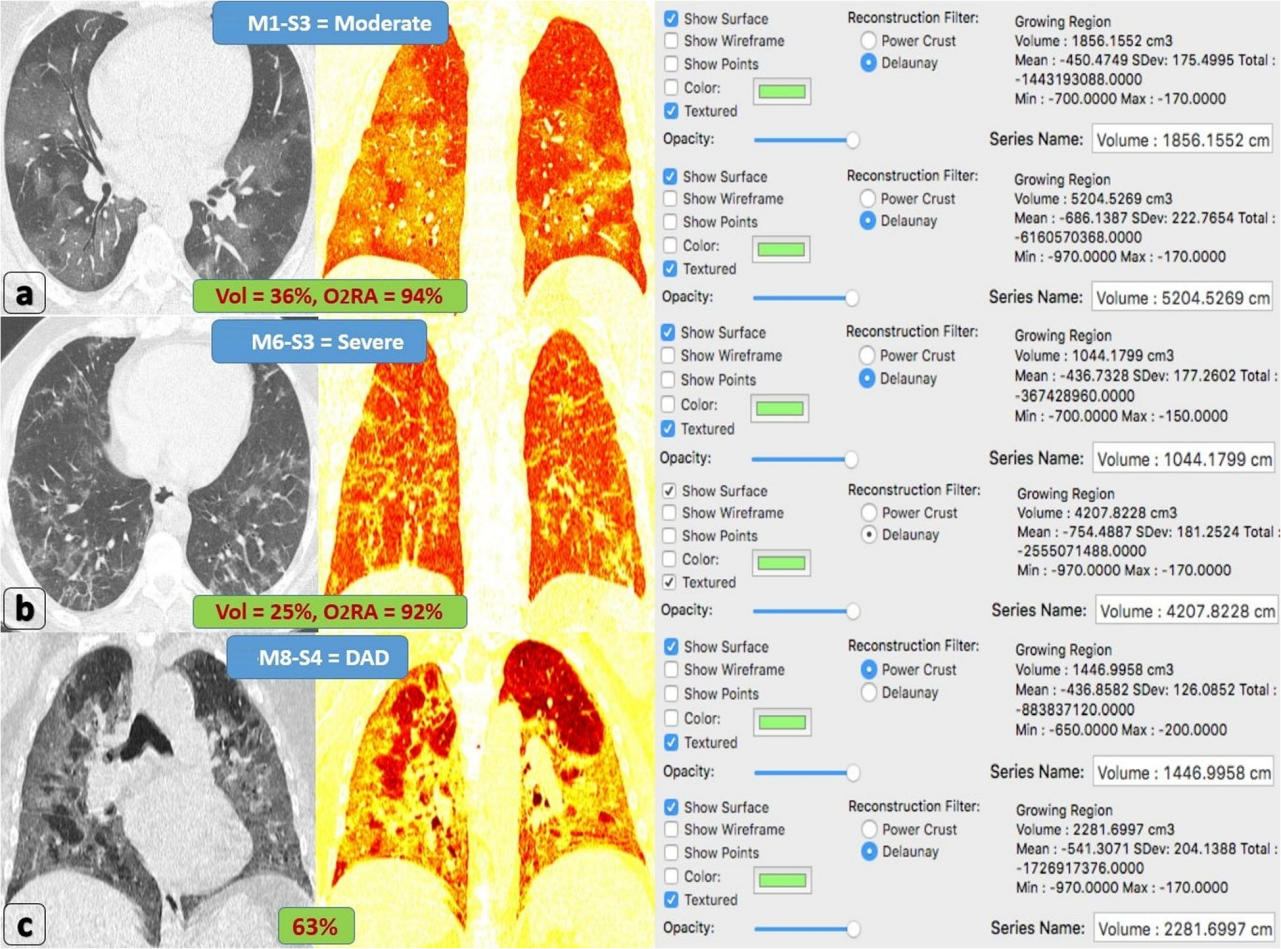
Combined morphologic/volumetric analysis. a A 33-year-old male patient proved with COVID-19 complaining of dyspnea, tachypnea, and 94% O_2_RA (consistent with border-line severity). Axial CT lung window and coronal 2D computed volumetric analysis showing bilateral widespread patchy pure GGOs. 3D computed volumetric analysis after threshold interval adjustment revealed 1856 cc pathological lung volume, 5204 cc total lung volume, and 36% lung involvement. Overall CT coding (M1-S3). b A 61-year-old male patient proved with COVID-19 complaining of dyspnea, tachypnea, and 92% O_2-_RA (clinically severe). Axial CT lung window and coronal 2D computed volumetric analysis show bilateral patchy GGOs mixed with early fibrotic changes and mild parenchymal distortion. 3D computed volumetric analysis after threshold interval adjustment revealed 1044 cc pathological lung volume, 4207 cc total lung volume and 25% lung involvement. Overall CT coding (M6-S3). c A 67-year-old male patient proved with COVID-19 complaining of severe dyspnea and 82% O_2_RA (critical patient indicated for intubation). Coronal CT lung window and coronal 2D computed volumetric analysis showing bilateral diffuse lung involvement with GGOs and super-added septal thickening “crazy-paving pattern.” 3D computed volumetric analysis after threshold interval adjustment revealed 1446 cc pathological lung volume, 2281 cc total lung volume, and 64% lung involvement. Overall CT coding: M8-S4 = DAD
